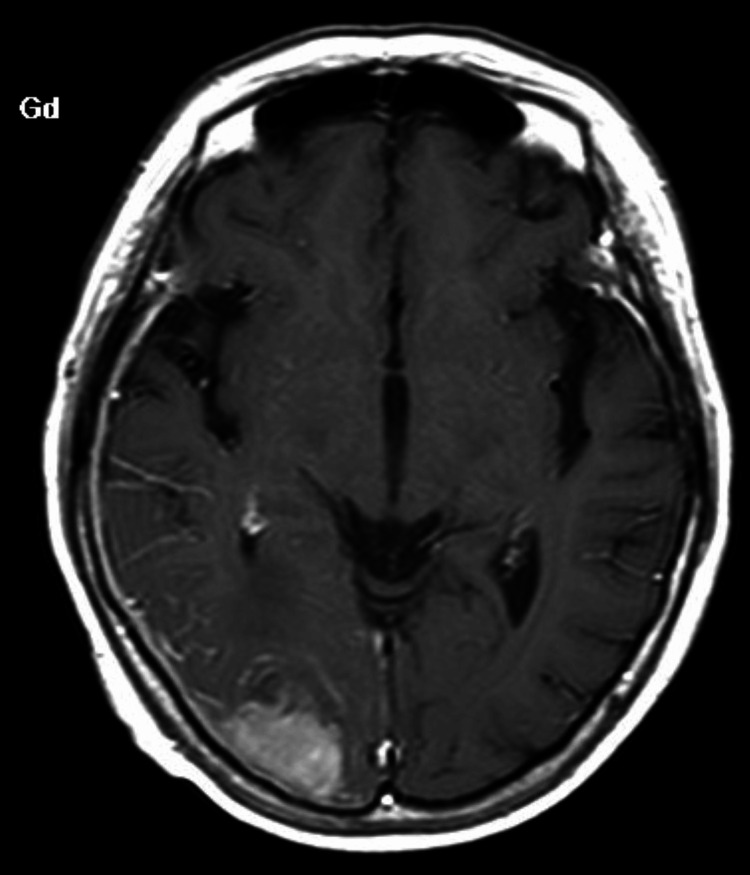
Contrast-enhanced brain MRI image at relapse reveals the mass showing heterogenous contrast enhancement; dural thickening is also observed from the right temporal area to the parietal area
